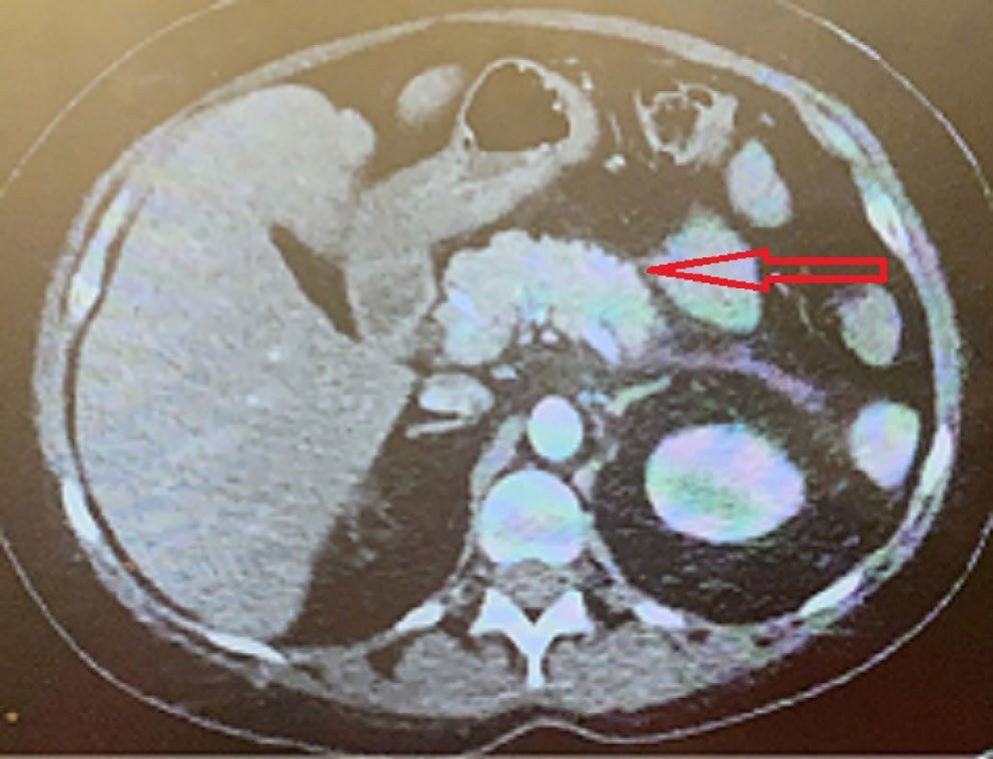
CT showing an inflamed and edematous pancreas as depicted by the arrow, concerning for pancreatitis
